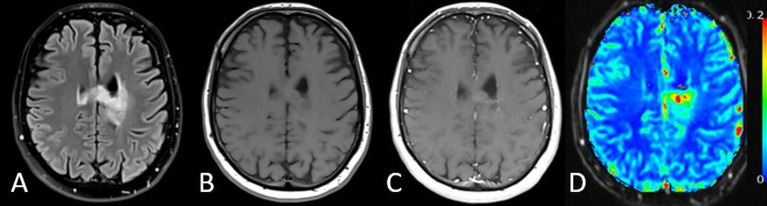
MRI results from a 32-year-old patient with a grade 2 oligodendroglioma. (A)
